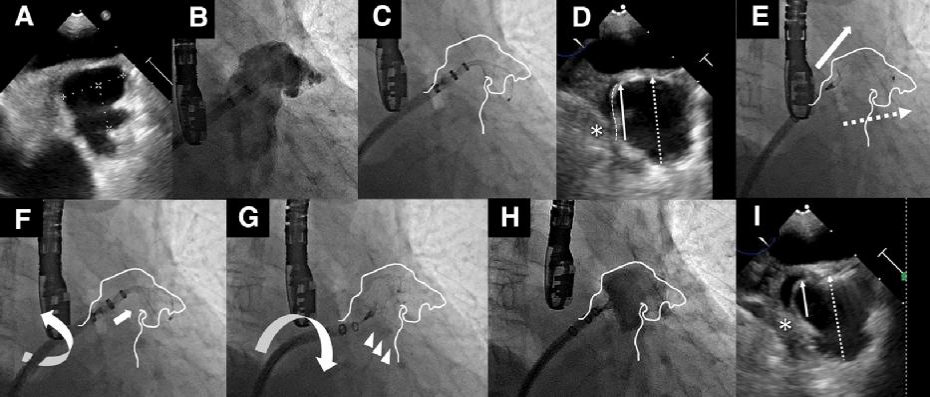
(*A*) Transoesophageal echocardiography (TEE) measurement of the maximum diameter of the left atrium appendage (LAA) orifice (25.0 mm); (*B*) baseline angiography; (*C*) the delivery sheath was placed in the anterior lobe, and the device was moved posteriorly; (*D*) in the TEE image, the extending device shoulder was represented by the white dot curve. TEE established the total length of device was calculated as 25.2 mm (white arrow dots), and the device's extension from the LAA ostium was measured at 16.9 mm (white arrow). Significant device protrusion of more than 50% from the LAA ostium was discovered along with a minor space (white asterisk) on the left side of device that did not meet the conventional device release criteria; (*E*) inadequate alignment between the delivery sheath (white arrow) and device (white arrow dots); video of the ‘cobra twist’ technique; (*F*) the delivery sheath was positioned using anticlockwise rotation (white arrow circle) and keeping the proximal device cobra-like head with forward pressure (white arrow); (*G*) the delivery sheath was then intentionally twisted clockwise (white circular arrow), which reduced the posterior device protrusion (white arrows); the process was deemed successful based on the results of (*H*) angiography and (*I*) TEE, which revealed that the device release criteria was <50% device protrusion from the LAA ostium [total device length: 25.5 mm (white arrow dots), protruded length: 12.0 mm (white arrow)] and no residual space in the left side of device (white asterisk).
